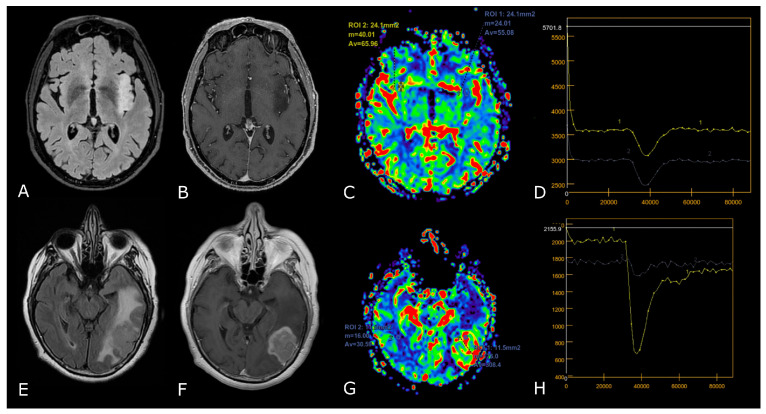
DSC-PWI in differentiating HGG from LGG. Axial T2-FLAIR (A) and post-contrast T1w (B) of a low grade IDH-mut left insular astrocytoma. DSC-PWI demonstrates normal rCBV (C) and complete return to baseline of the signal-intensity-time curve (D). The second row shows axial T2-FLAIR (E) and post-contrast T1w (F) of a left temporal-occipital GB. DSC-PWI demonstrates increased rCBV (G) and reduction of PSR consistent with BBB breakdown (H).
